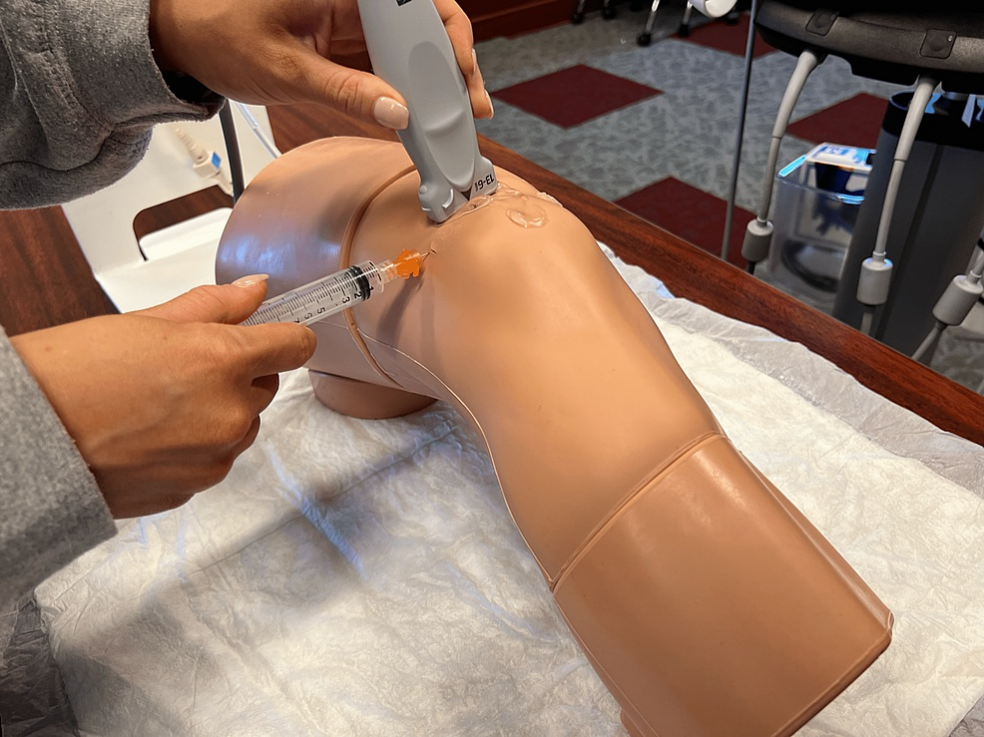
Demonstration of ultrasound-guided knee aspiration
